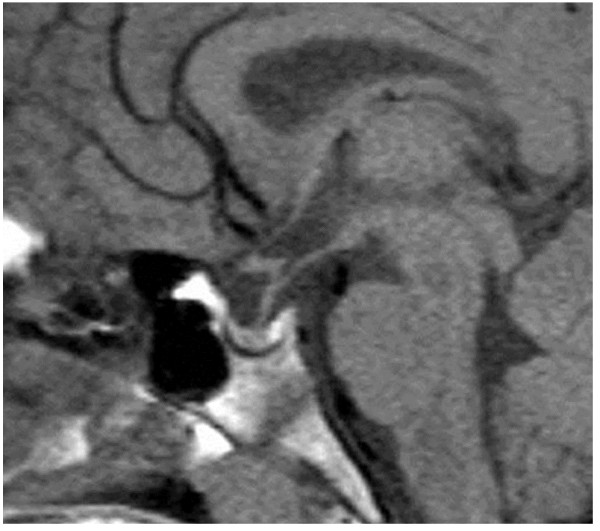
Magnetic resonance imaging scan of the pituitary gland of our patient.
